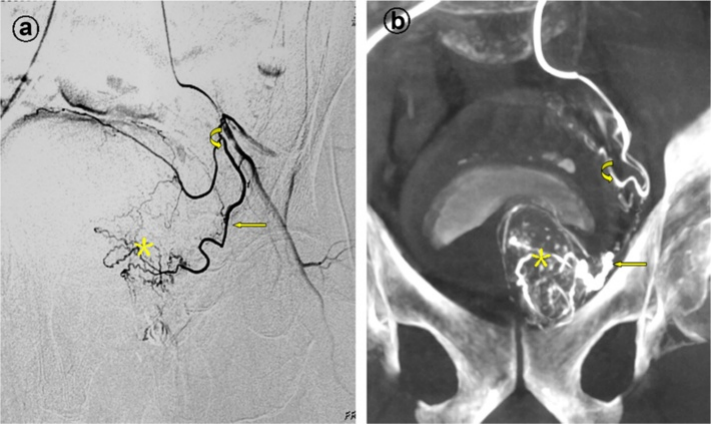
Prostatic artery arise from the superior vesical artery. Image from a patient with lower urinary tract symptoms due to benign prostatic hyperplasia (121 mL) underwent PAE. a. Digital subtraction angiography (DSA) of the anterior division of the left internal iliac artery with ipsilateral oblique view demonstrates the left prostatic artery (straight arrow) and the superior vesical artery (curved arrow). The asterisk indicates the corkscrew pattern of intra-prostate arteriola. b. Cone-beam CT image with coronal view after selective catheterization of the anterior division of the left internal iliac artery demonstrates the left prostatic artery (straight arrow) and the superior vesical artery (curved arrow). The asterisk indicates the corkscrew pattern of intra-prostate arteriola.
